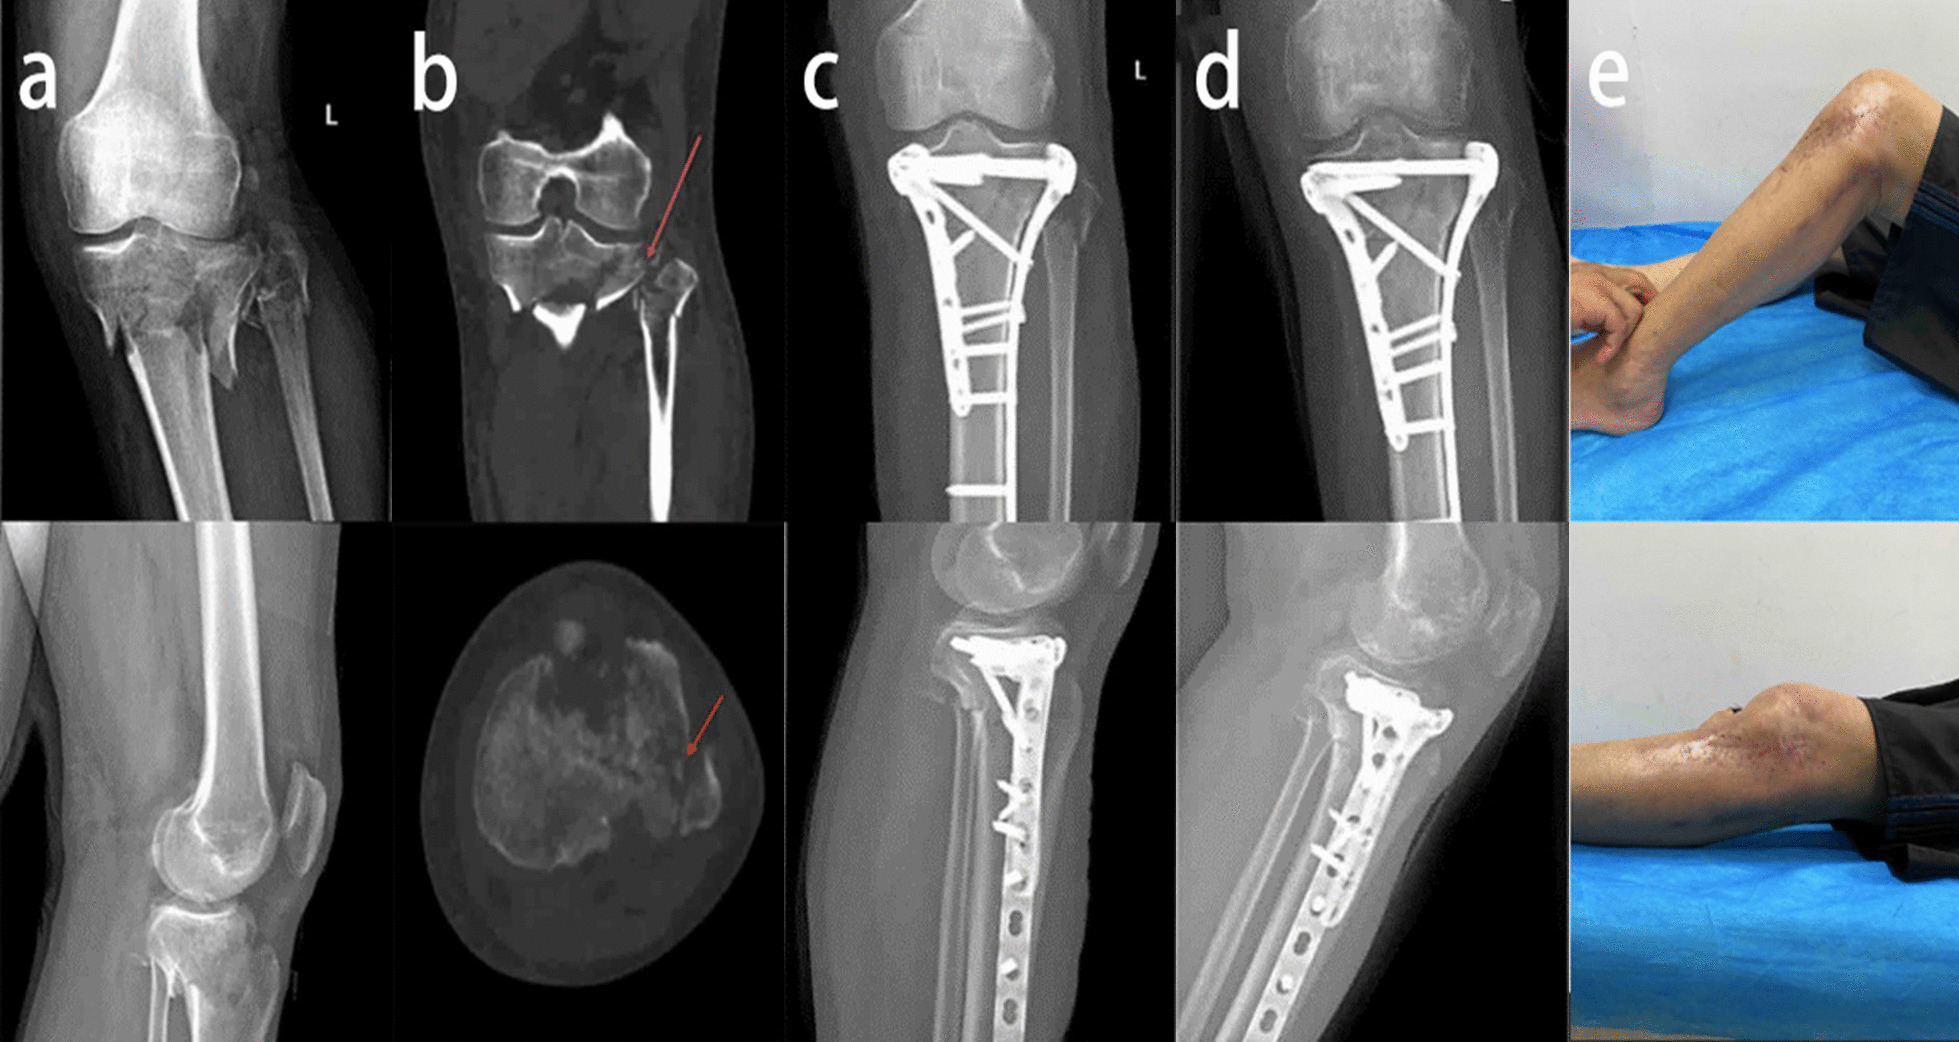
a Preoperative anteroposterior and lateral radiographs of a 38-year-old male patient. b CT scan indicating fractures of the proximal fibula and PJF (red arrow). c Postoperative anteroposterior and lateral radiographs. d Anteroposterior and lateral radiographs indicated good fracture healing six months after the operation. e Slightly limited flexion function
